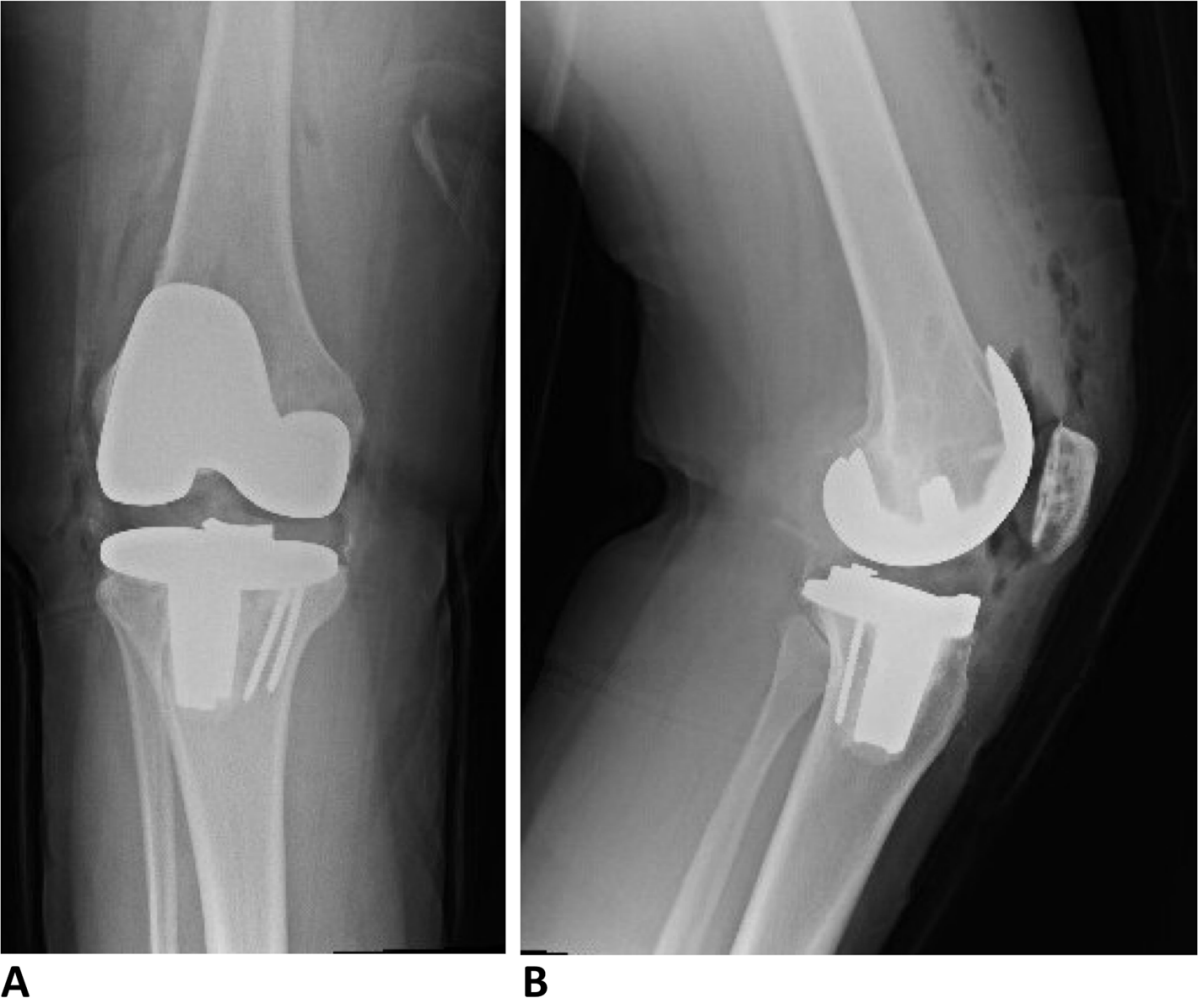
Postoperative radiographs demonstrating appropriate alignment and position of total knee arthroplasty implants on (a) AP view and (b) lateral view
